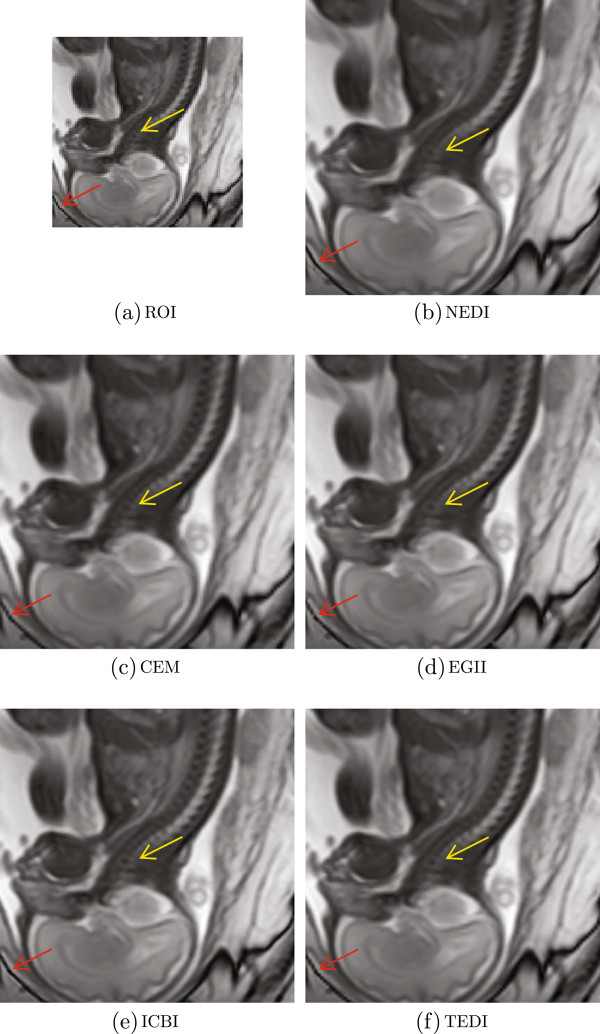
Visual analysis of structures in ROI. (a) is original fetal spine MR image, (b-f) stand for the interpolated results from NEDI, CEM, EGII, ICBI, and TEDI, respectively. Because of low quality in (a), unnatural textures, loss of edge sharpness and errors are seen. Interpolated result from TEDI shows better consistency with the original image.
